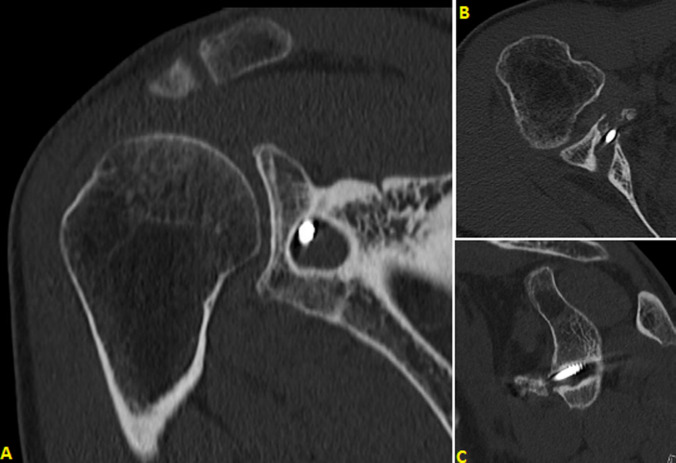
(A,B,C) CT scan of right shoulder; axial, coronal and sagittal slices
